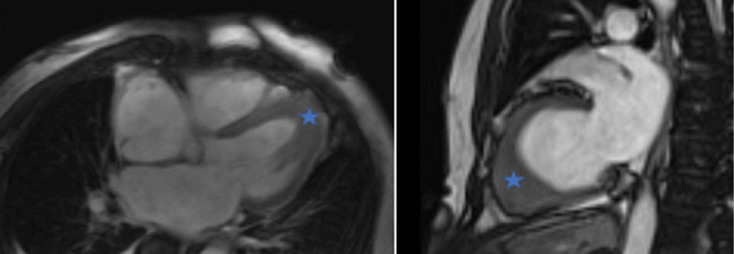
cardiac MRI demonstrating focal apical left ventricular hypertrophy (star), confirming the diagnosis of apical hypertrophic cardiomyopathy
